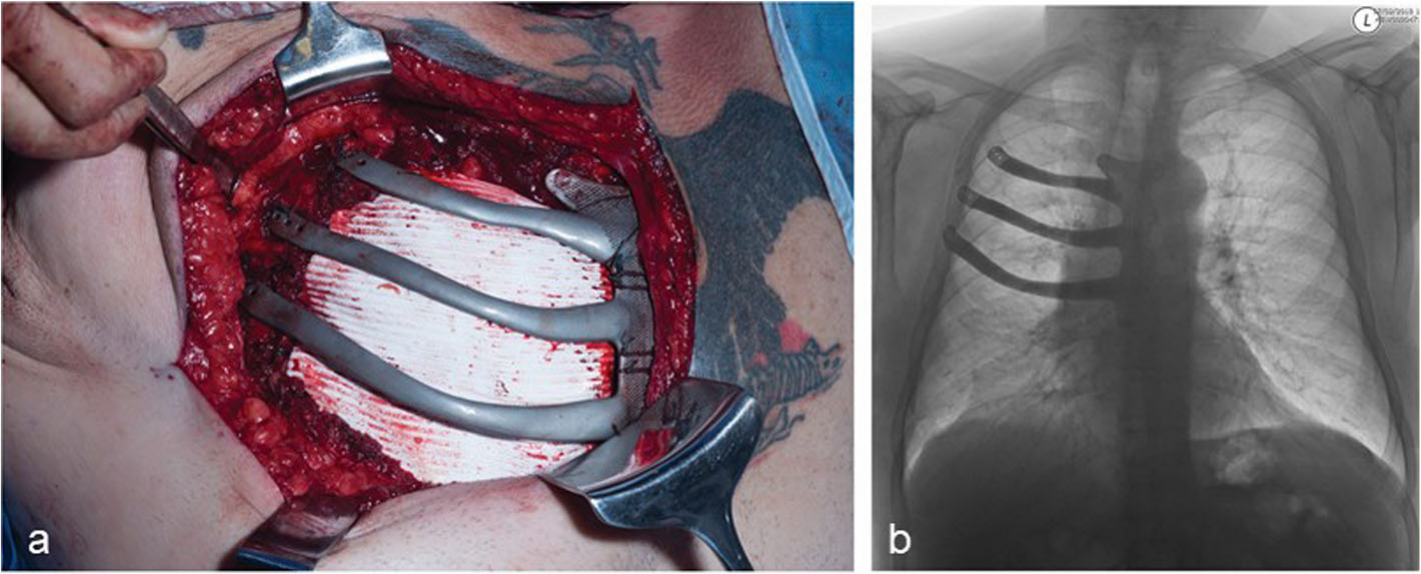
The implant securely in place at surgery (a) and on the follow-up chest radiograph (b)
